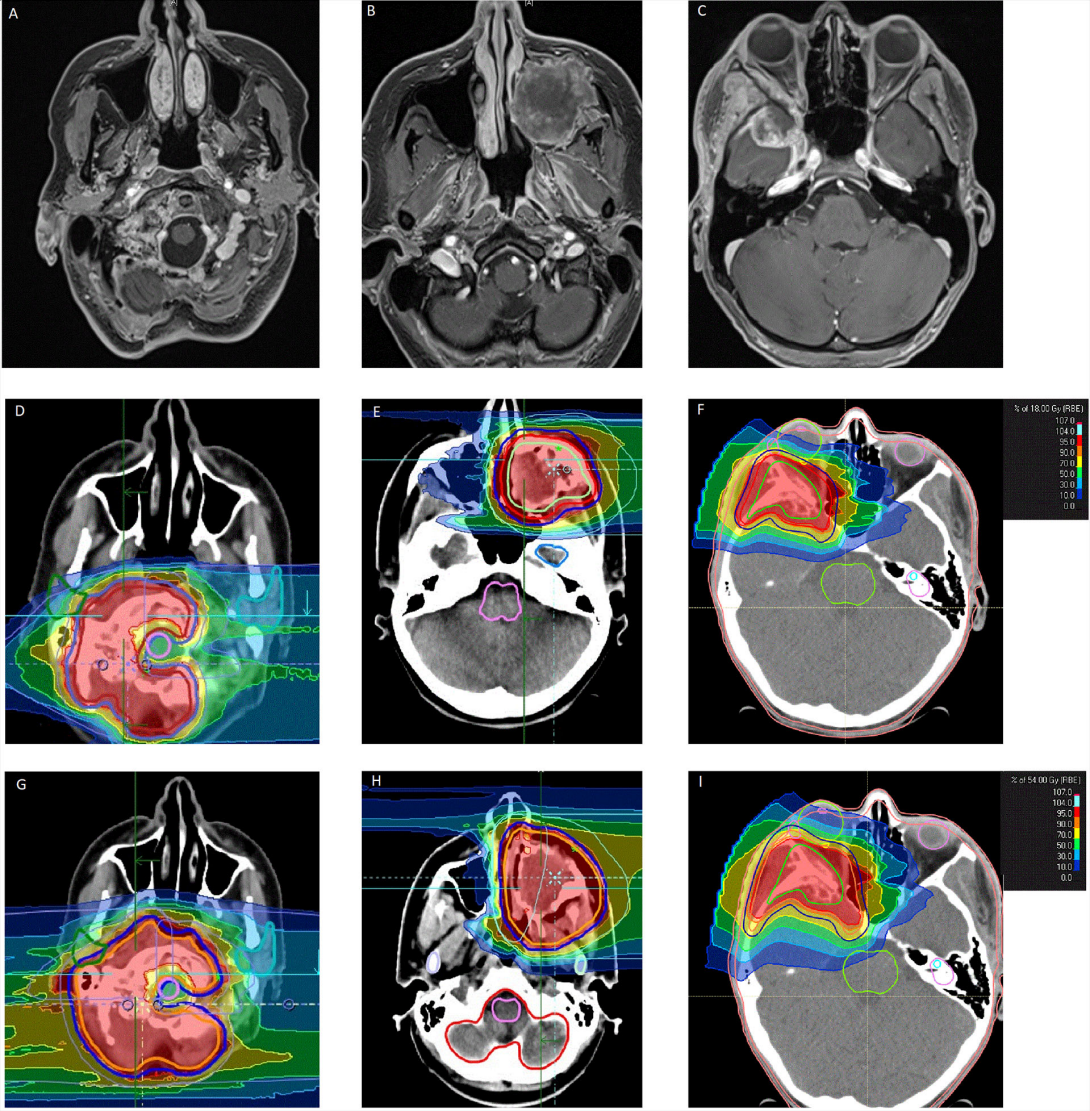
Examples of craniofacial osteosarcoma treated by combined ion-beam radiotherapy. (A–C) Representative contrast-enhanced T1 MRI sequences of inoperable craniofacial osteosarcoma. (D–F) Target volume delineation and dose distribution of the according carbon ion boost (18 GyRBE in six fractions). (G–I) Target volume delineation and dose distribution of the according proton base boost (54 GyRBE in 27 fractions).
